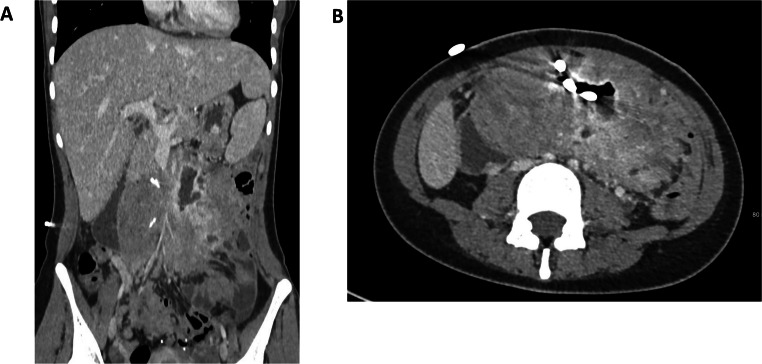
CT A coronal and B axial images – diffuse mesenteric desmoid with small bowel fistulas and central cavity with drain in situ
